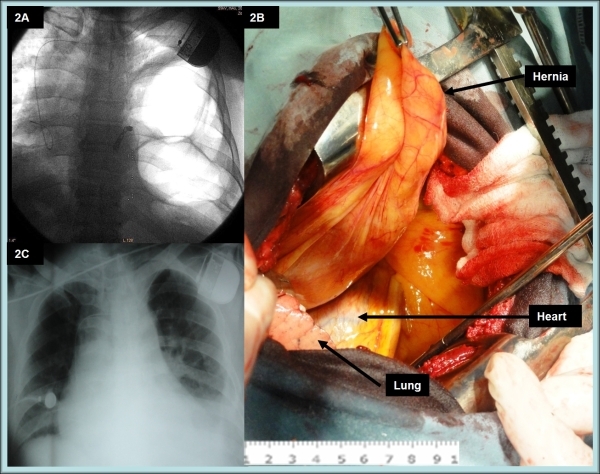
2A, Initial chest fluoroscopy shows the permanent pacemaker with the pacing lead in right atrium and hypo dense (bowel density) mass in the left chest cavity. 2B, Herniated abdominal viscera into the thorax. Heart and lung tissue are also seen after thoracotomy. 2C, Chest X-ray after surgical repair: normal anatomical position of chest organs.
